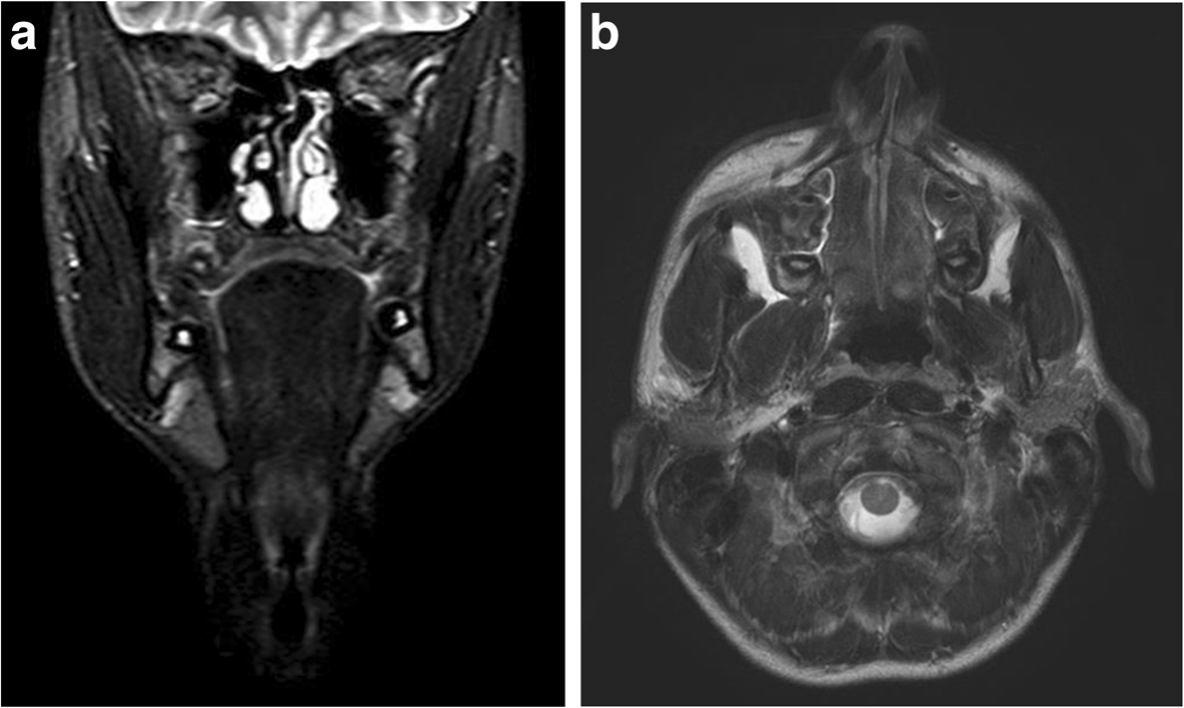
MRI of Progressive Hemifacial Atrophy. a: Coronal view from MRI demonstrating left hemifacial atrophy of soft tissue and osseous structures. b: Axial view from MRI demonstrating left hemifacial atrophy of soft tissue and osseous structures.
